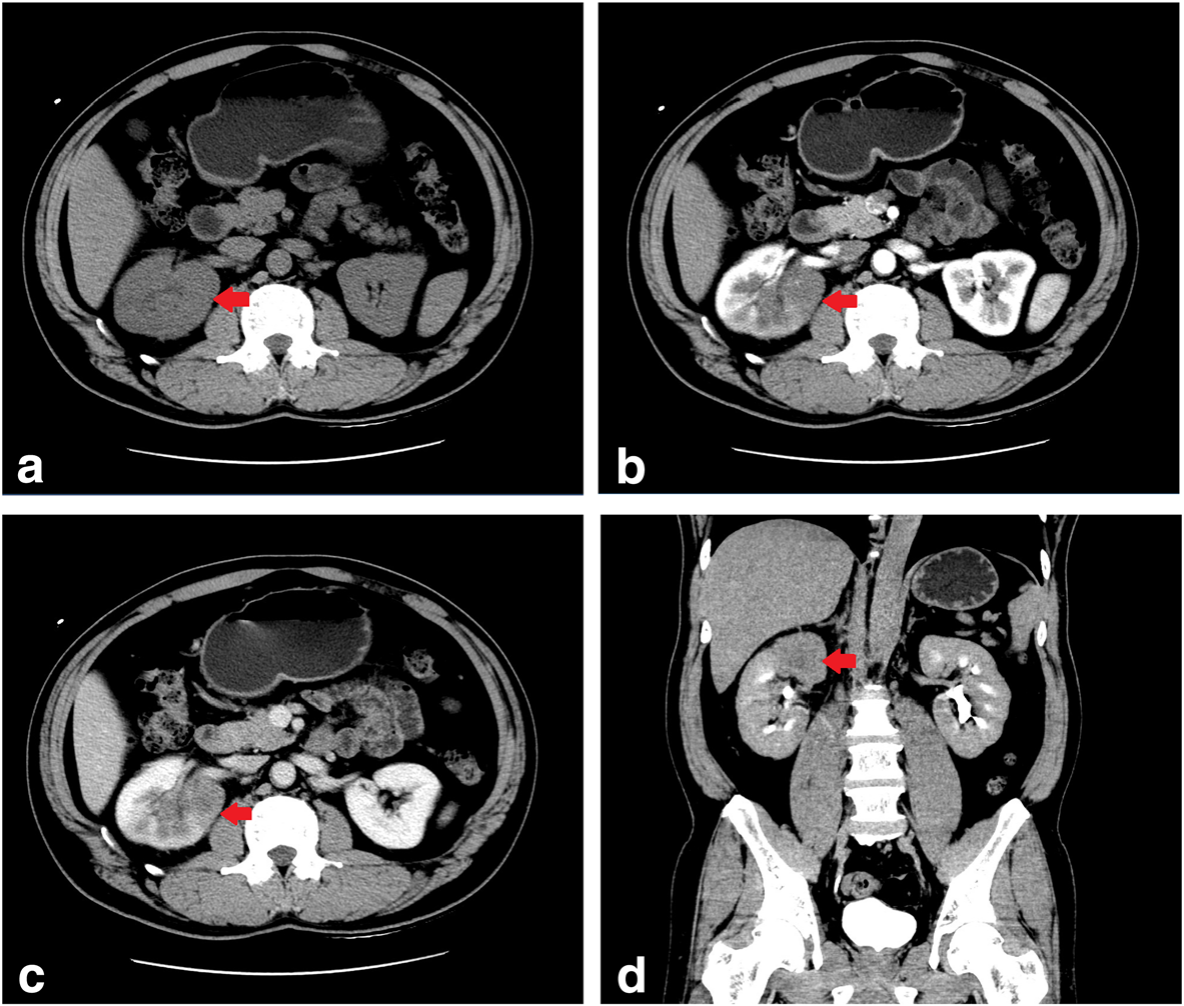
A computed tomography (CT) urogram was performed. Axial and coronal computed tomographic images of the abdomen demonstrating a 6.0 × 4.7 cm irregular mass centered within the superior pole of the right kidney. On the axial noncontrast image (a), the mass is non-homogeneous with the most portions being isodense to the normal renal parenchyma and with central hypoattenuating areas suggestive of necrosis. During the corticomedullary phase (b), the nephrographic phase (c) and the coronal excretory phase (d), the solid component of the mass mild-moderately enhances but not as avidly as normal renal cortex. The central low density necrotic areas and the margin become more conspicuous
